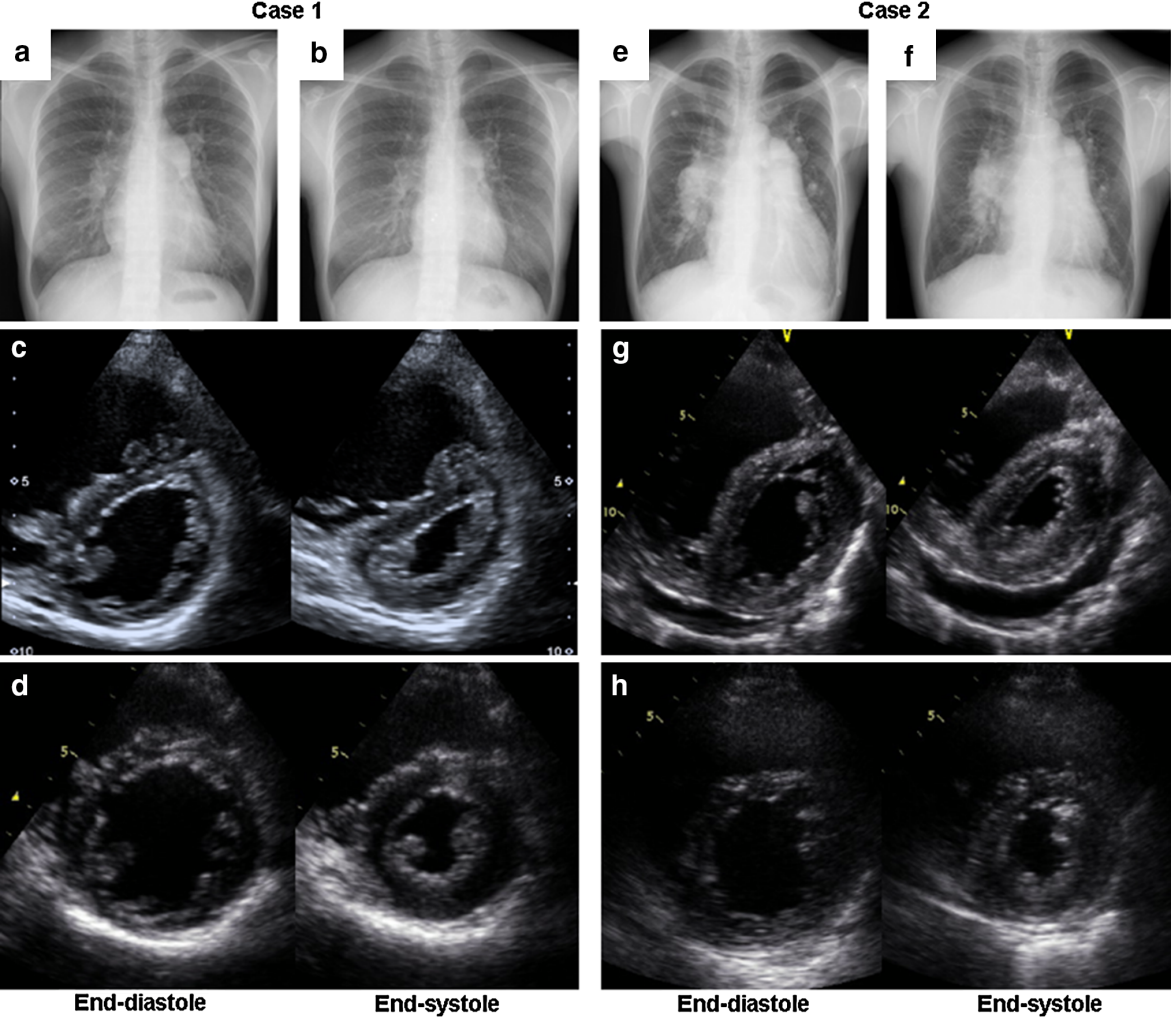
Chest X-ray and trans-thoracic echocardiogram. Case 1 (a–d) and case 2 (e–h). Cardiomegaly and ventricular overload were dramatically improved after closure (b, d, f, h) compared with those on admission (a, c, e, g)
